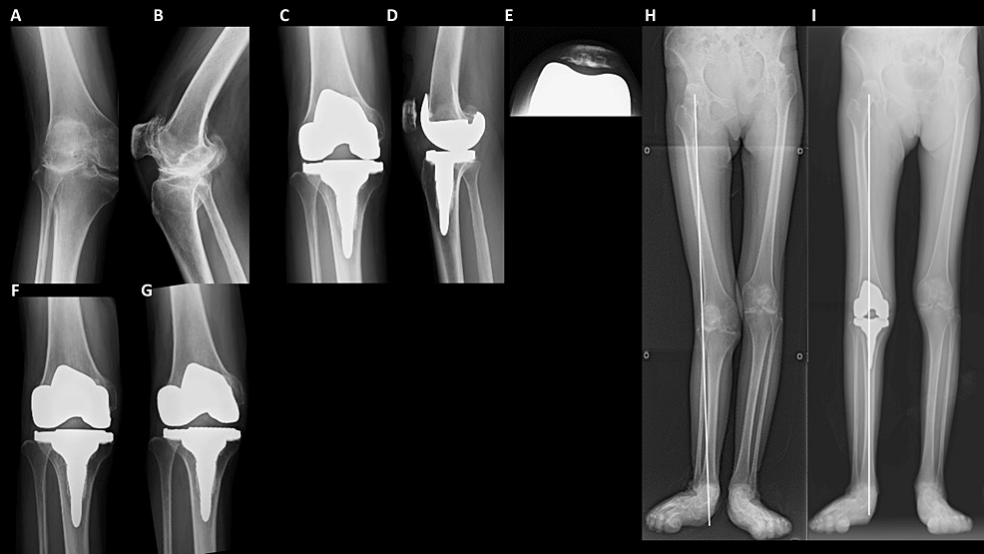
Radiographs of a representative case presentation A 48-year-old woman with a 22-year history of RA (Steinbrocker classification: stage IV class III) had a total 2011 KSS of 44 points (full score, 180 points). She could walk with a T cane for only a few minutes. (A) Preoperative radiograph of the anterior weight-bearing view. (B) Preoperative radiograph of the lateral weight-bearing view. (C) Postoperative radiograph of the anterior weight-bearing view. (D) Postoperative radiograph of the lateral weight-bearing view. (E) Postoperative radiograph of knee skyline view. The patellar position was good. (F) Antero-posterior view under varus stress. (G) Antero-posterior view under valgus stress. There was almost no instability under varus/valgus stressed condition. (H) Preoperative radiograph of the whole lower extremity. The white line shows the loading axis of the whole lower extremity. (I) Postoperative radiograph of the whole lower extremity. The passing point of the loading axis in the knee joint was centralized. RA, rheumatoid arthritis; KSS, Knee Score System
